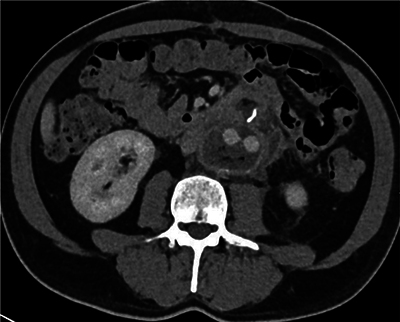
Axial CT scan showing the présence of gaz in the aneurysmal sac.
